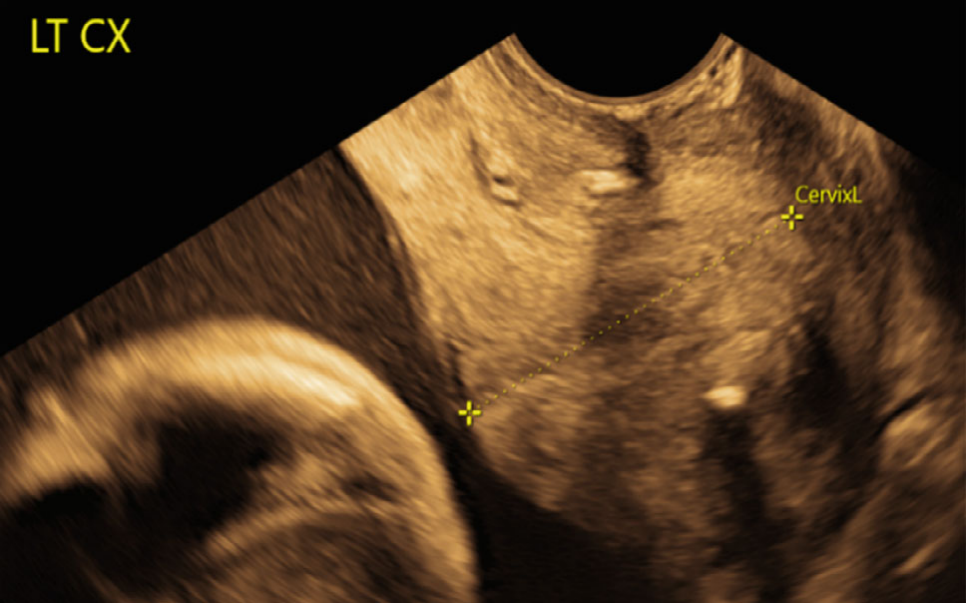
Two weeks post‐cerclage placement follow‐up transvaginal ultrasound demonstrating 35.5 mm functional residual cervix and intact cerclage placement (left cervix).
